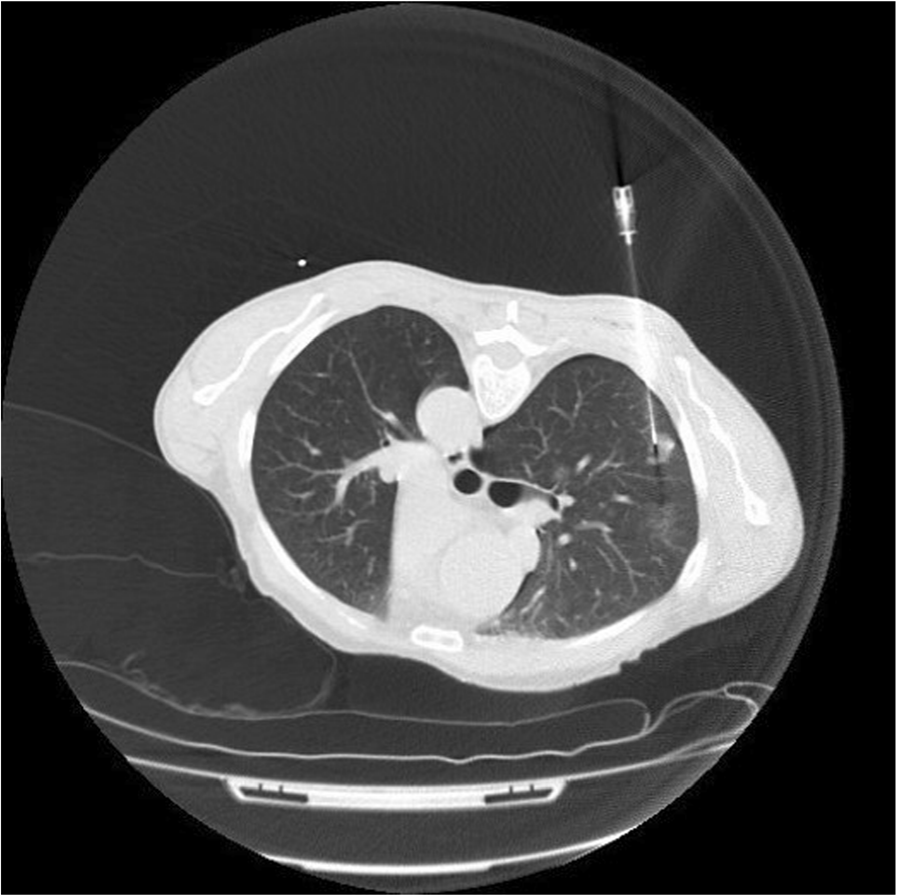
CT-guided biopsy of a part-solid nodule in the right upper lobe. Every effort should be made to target the solid component of the lesion which correlates with the invasive component of the nodule. The biopsy has been complicated by a small pneumothorax
